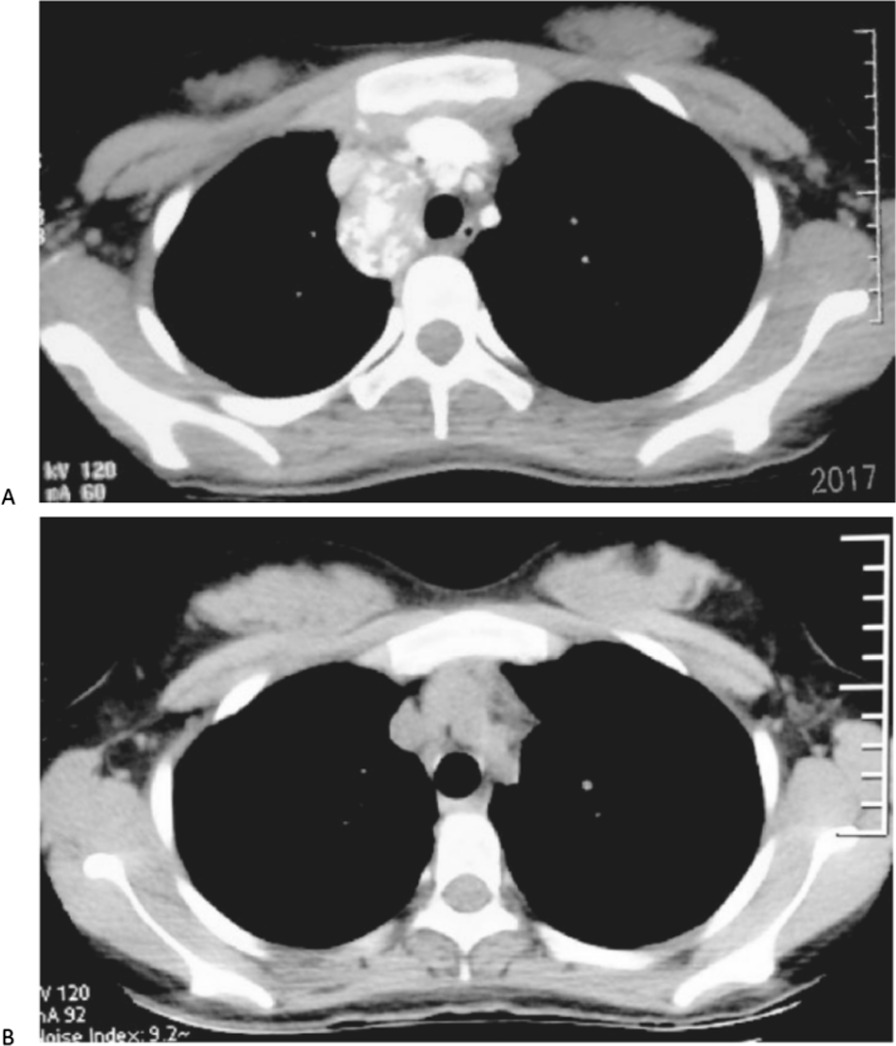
A 15-year-old patient with recurrent MTC. A Preoperative findings of suspicious lymph nodes in the right upper mediastinal compartments upon enhanced CT scan. B Postoperative image 3 years after video mediastinoscopy-assisted superior mediastinal dissection and central and lateral neck dissection was performed
